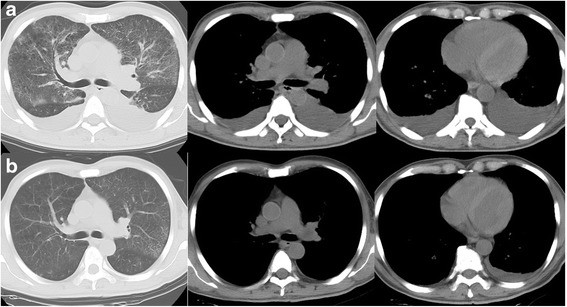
Computed tomography of the chest. a Computed tomography radiograph showing ground-glass opacities in the upper lungs with moderate pleural effusions, and findings suggestive of right ventricular pressure overload (enlargement of the right ventricle and main pulmonary artery). b Computed tomography radiograph at 4 weeks after admission showing resolution of the abnormal lung shadows along with signs of alleviation of the right ventricular pressure overload. These helical computed tomography images consisted of 5-mm collimation sections, with window settings appropriate for viewing both the lung (window level from −700 HU, window width from 2000 HU) and the mediastinum (window level from 40 HU, window width from 400 HU)
